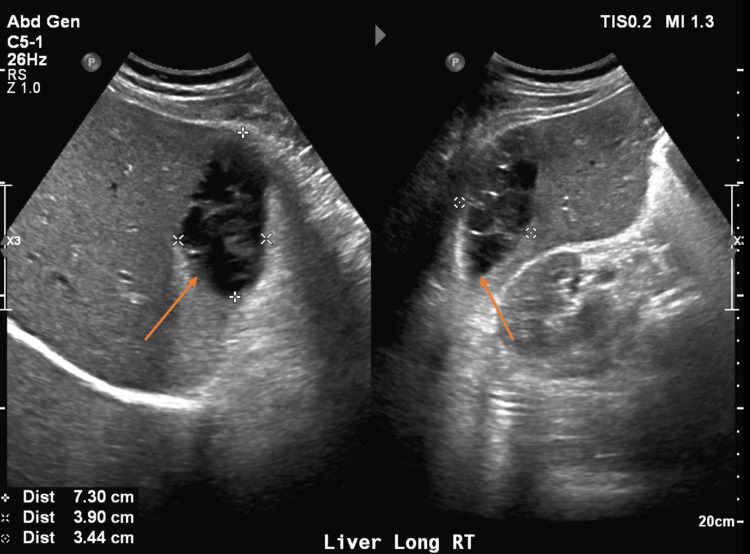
Abdominal ultrasound demonstrating an area of mixed echogenicity on the right side of the liver measuring 7.3 cm × 3.9 cm, likely a hematoma or an abscess (arrow).
